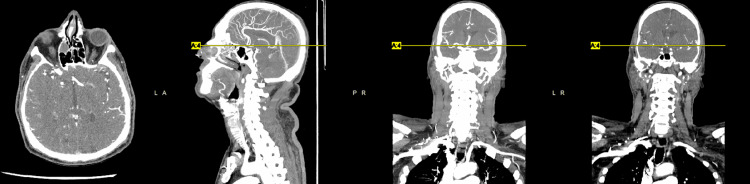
CT angiography of the brain showing no acute large vessel occlusion.
